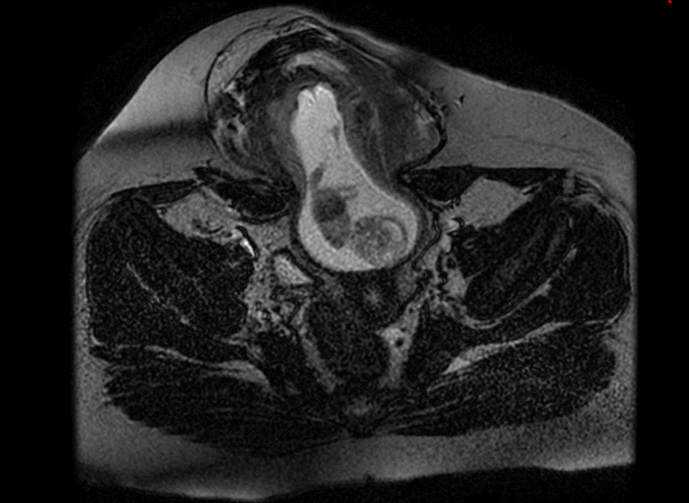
transversal cut:MRI show strangulation of a pregnant uterus through an orifice of 6 cm, resulting in a strangulated hernia of a pregnant uterus at the umbilical level, with.
